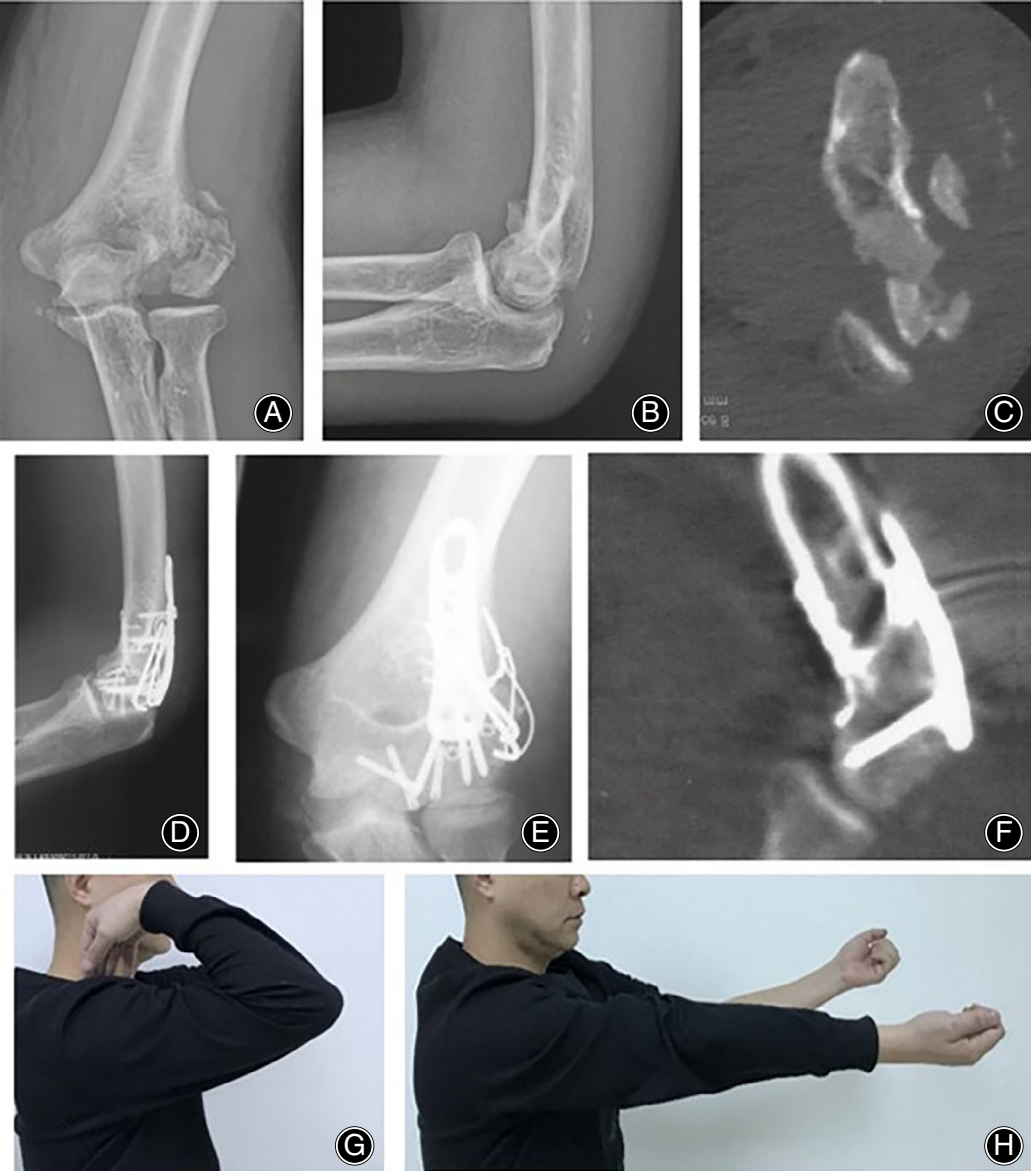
A 36‐year‐old male patient with capitellar fractures (Dubberley IIIB type) and avulsion fracture of the triceps brachii junction. Patient was treated with opening and micro‐locking plate parallel fixation 6 days after injury. (A–C) Preoperative anteroposterior and lateral X‐ray and CT scan showing frontal plane fracture of humerus. (D–F) CT and X‐ray scans 2 days after surgery showing satisfactory reduction and internal fixation. (G, H) Satisfactory recovery of elbow function 1 year after operation.
